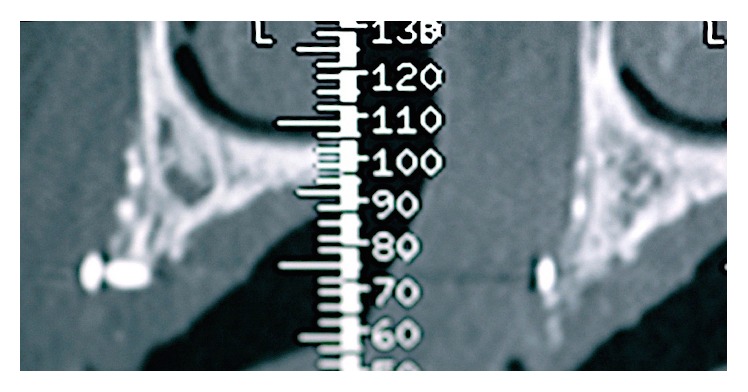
Cross-sectional CBCT scan showing the residual graft material and the cortical screw more coronally.
